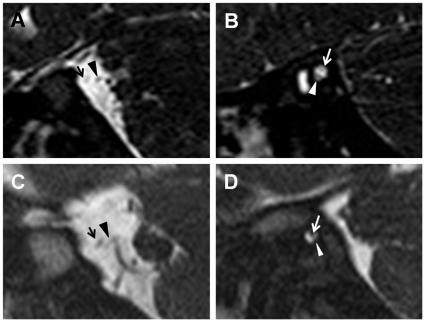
The parasagittal images of temporal MRI in patients with CHARGE syndrome. (A–B) The cochleovestibular nerve (arrowheads) is larger in diameter than the facial nerve (arrows) at the cerebellopontine angle (A) and within the internal auditory canal (B) in Patient 1. (C–D) The cochleovestibular nerve (arrowheads) is smaller in diameter than the facial nerve (arrows) at the cerebellopontine angle (C) and within the internal auditory canal (D) in Patient 4.
